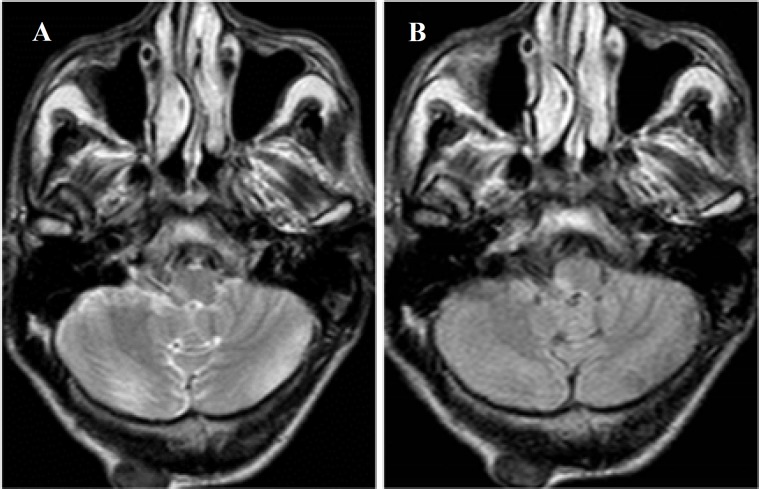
Brain magnetic resonance angiography (MRA) in T2WI (A) and FLAIR (B) revealed hypersignal lesion in the right medulla.
